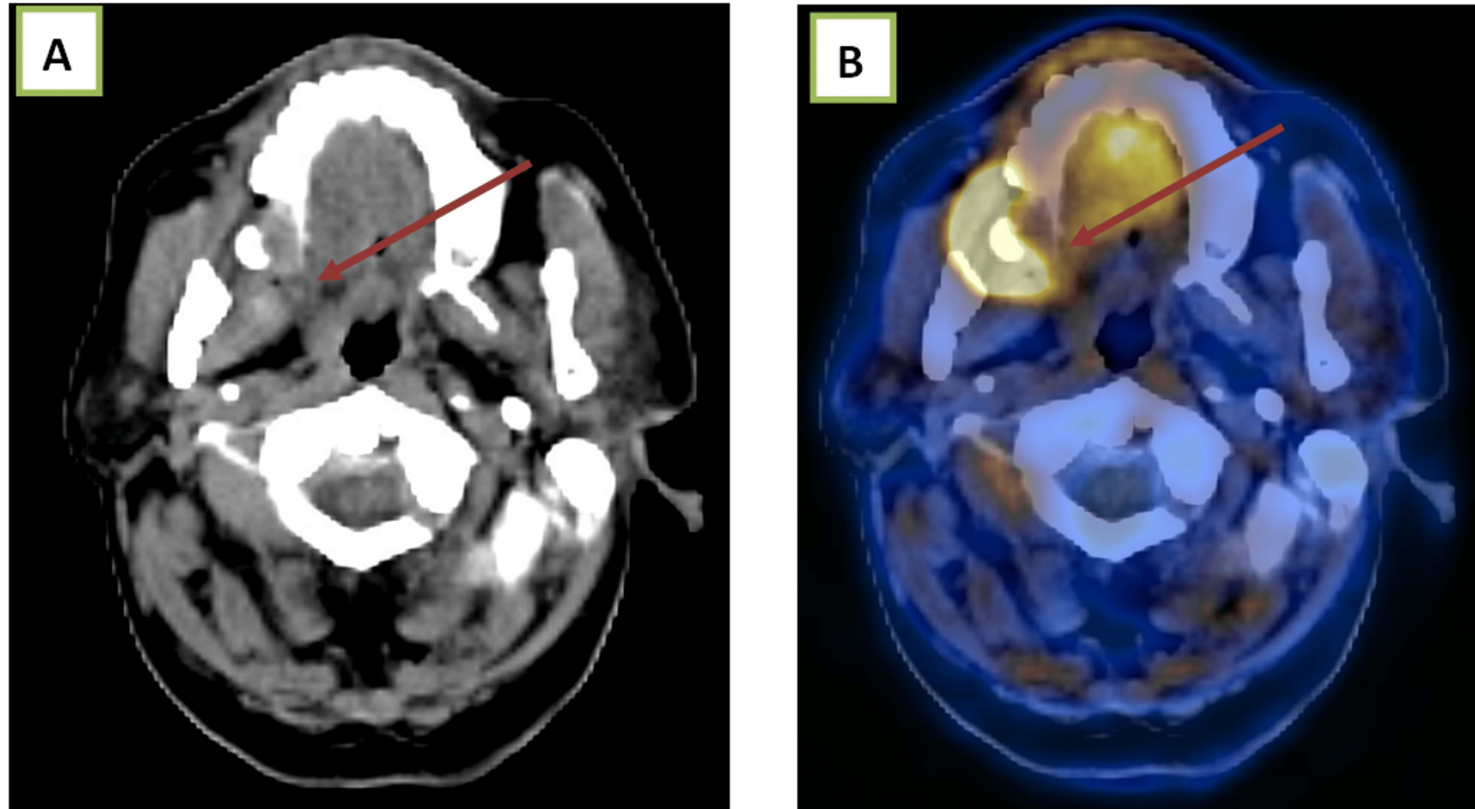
(A) Contrast-enhanced CT and (B) fused PET-CT images show a heterogeneously enhancing lesion in the right retromolar trigone and hard palate, with significant uptake on the fused PET-CT image. PET-CT, positron emission tomography-computed tomography
